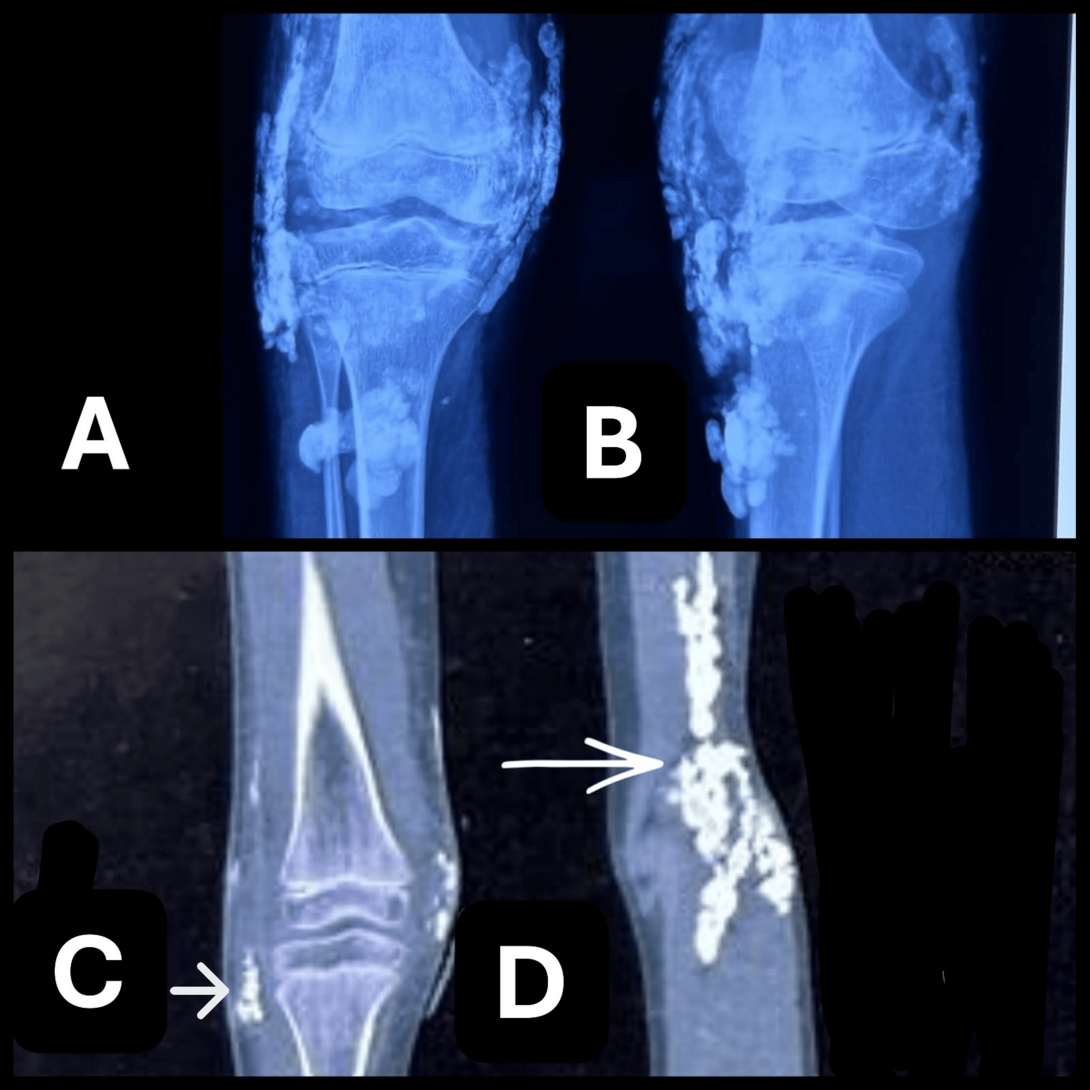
Dystrophic calcification (A) AP and (B) lateral radiographs of the left knee showing overall extensive calcification. Coronal cut computed tomography of the (C) right and (D) left knee showing calcification (white arrows).
